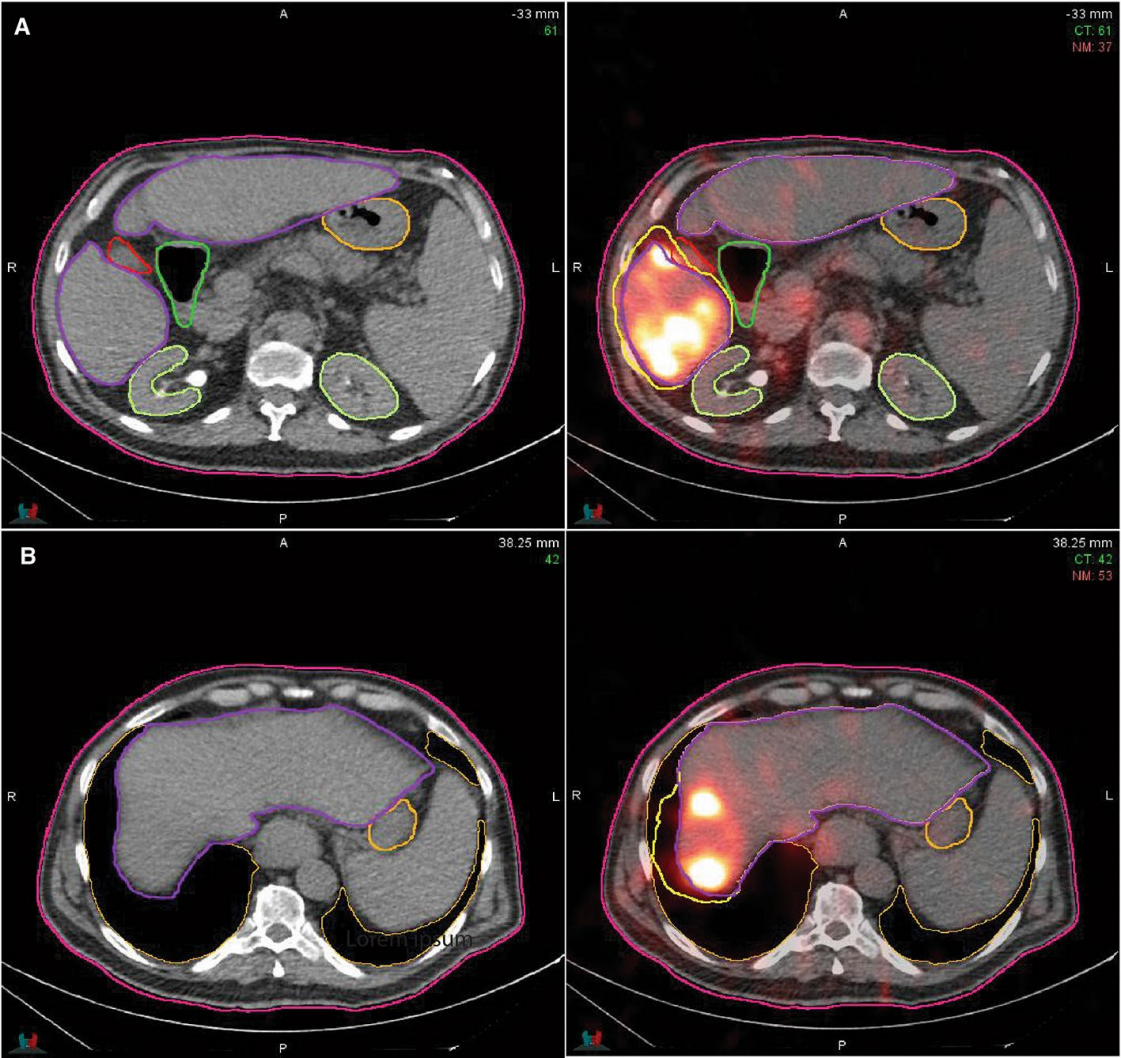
Contour of two axial slices for one patient are visualized where the left represents the CT and the right represents a SPECT/CT-fused image. The purple contour represents the original liver contours whereas the yellow represents the correction contour that includes valid, but misplaced activities either due to patient motion or partial volume effects
